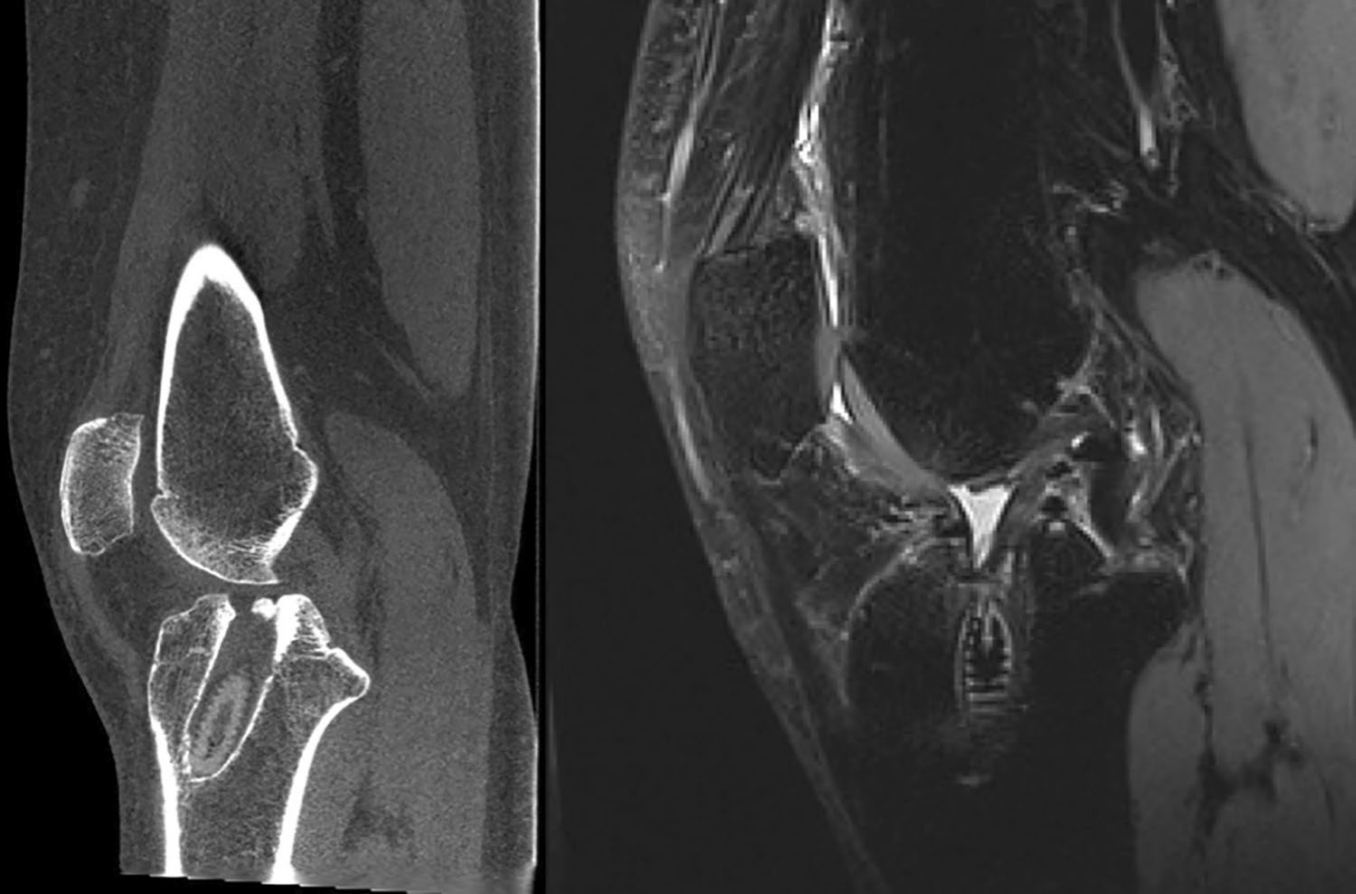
Pre-operative sagittal CT-Scan (on the left) and MRI (on the right) of a re-revision surgery case showing enlargement of the previous tibial tunnel
